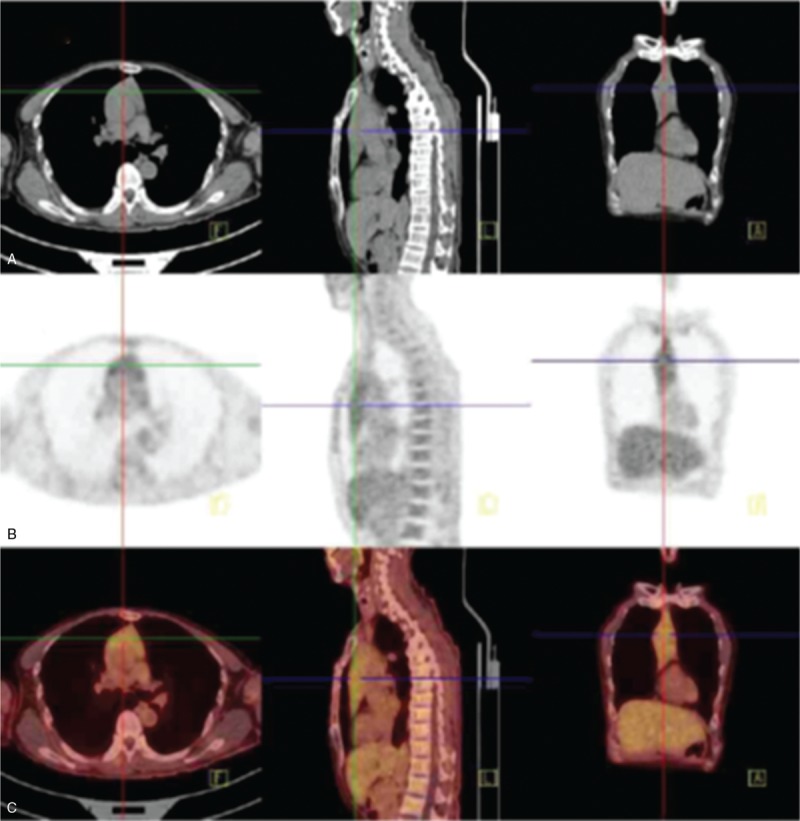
Positron emission tomography-CT (PET-CT) scan showing increased fluorodeoxyglucose uptake in an anterior mediastinal mass with relation to the thymus. CT (A), PET (B) and PET/CT-fused (C) images confirming the focal 18F-FDG uptake. 18F-FDG = 18F-fluorodeoxyglucose.
